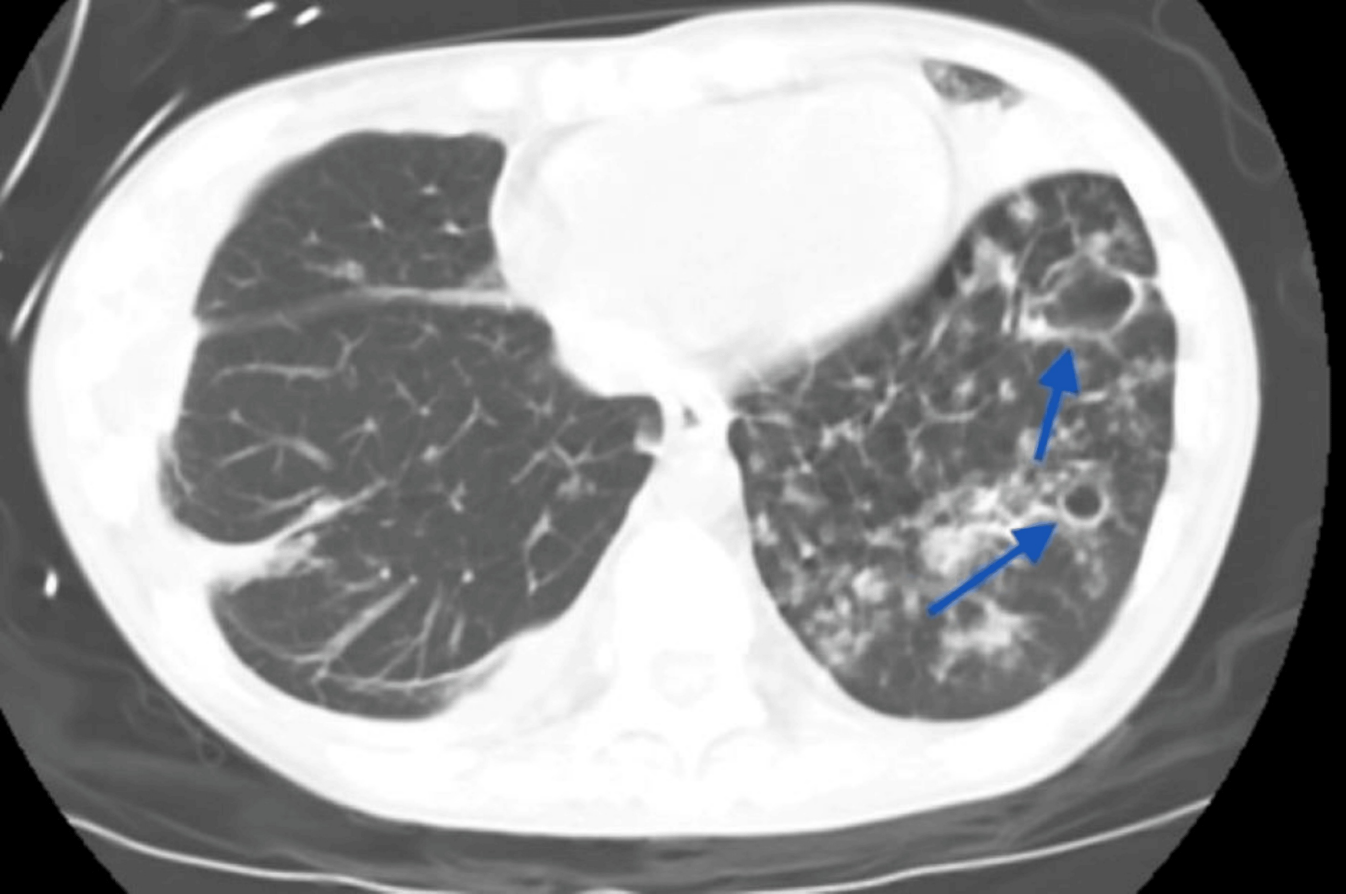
CT of the abdomen and pelvis revealing bibasilar reticulonodular opacities and left basilar cavitary lesions (blue arrows).
